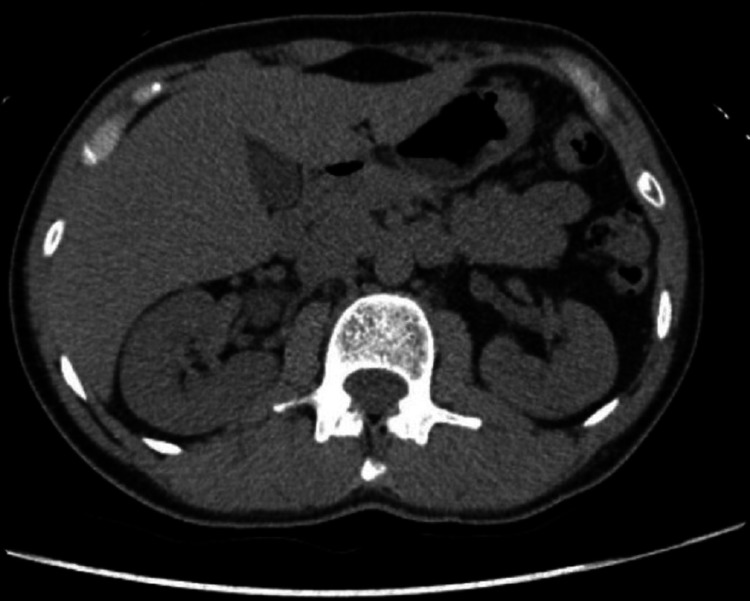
CT scan of the abdomen showing that both kidneys are in normal size and position, and the urinary tract is not obstructed. CT, computed tomography
